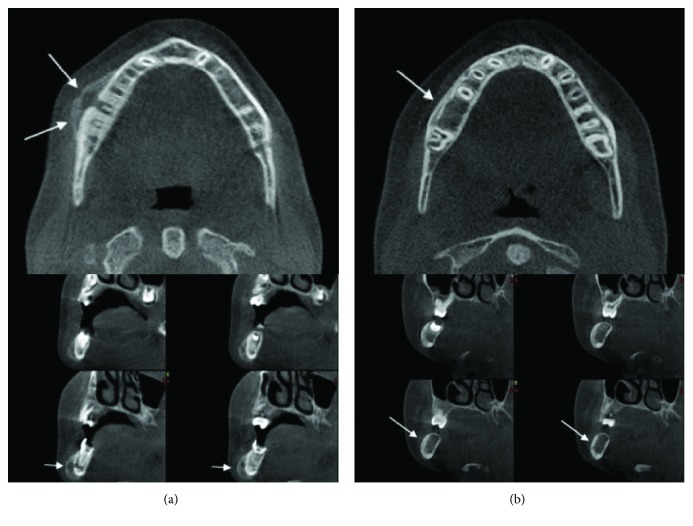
Axial and cross sections in CBCT showing new bone formation and a tunnel-like defect in the vestibule cortical surface of the inflamed bone starting from the apical region of tooth number 46 (a). CBCT image showing decreased cortical bone thickness and the presence of the original cortex within the enlarged portion of the jaw in the postoperative control (b).
